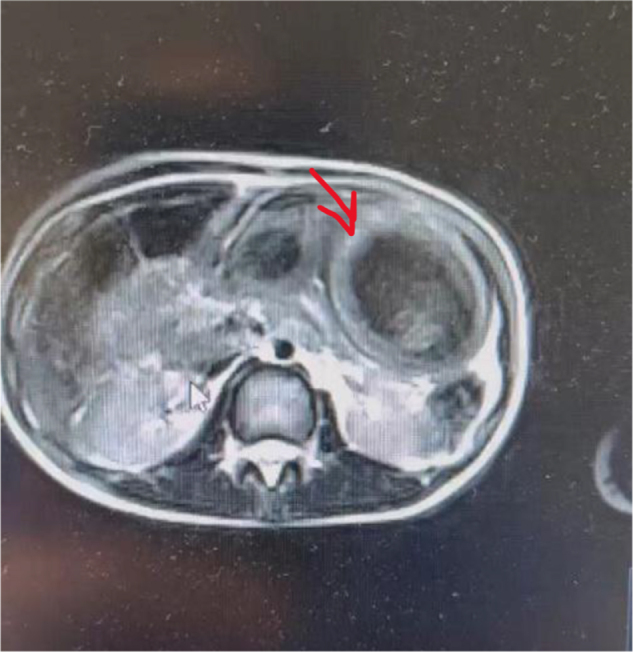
Axial MRI view confirming the intragastric location and internal heterogeneity of TBZ. The mass appears mottled and low signal intensity.
